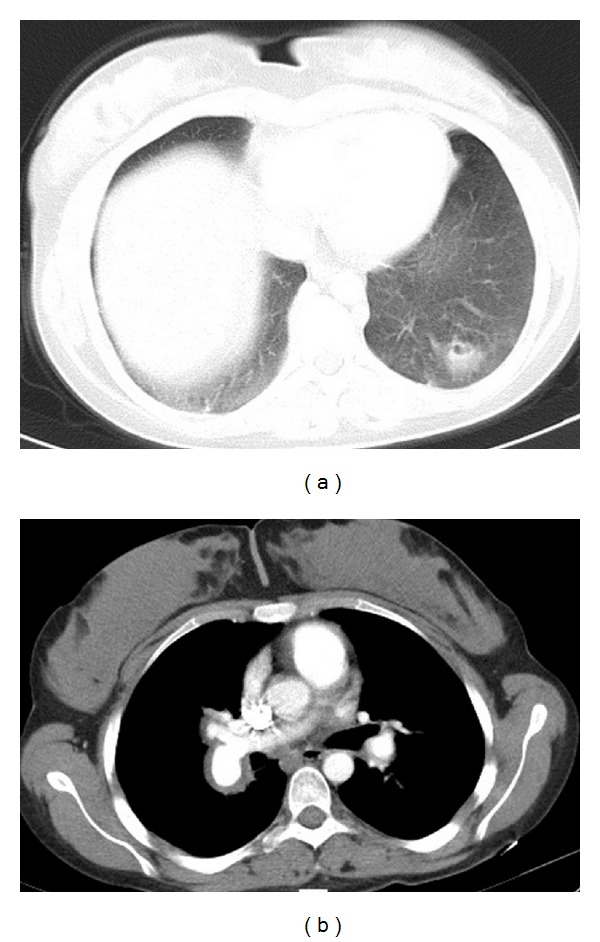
Minimisation of the consolidated substance related to the emboli on CT after the treatment (a), partial dissolution of the thrombus and minimisation of its PA diameter (b).
